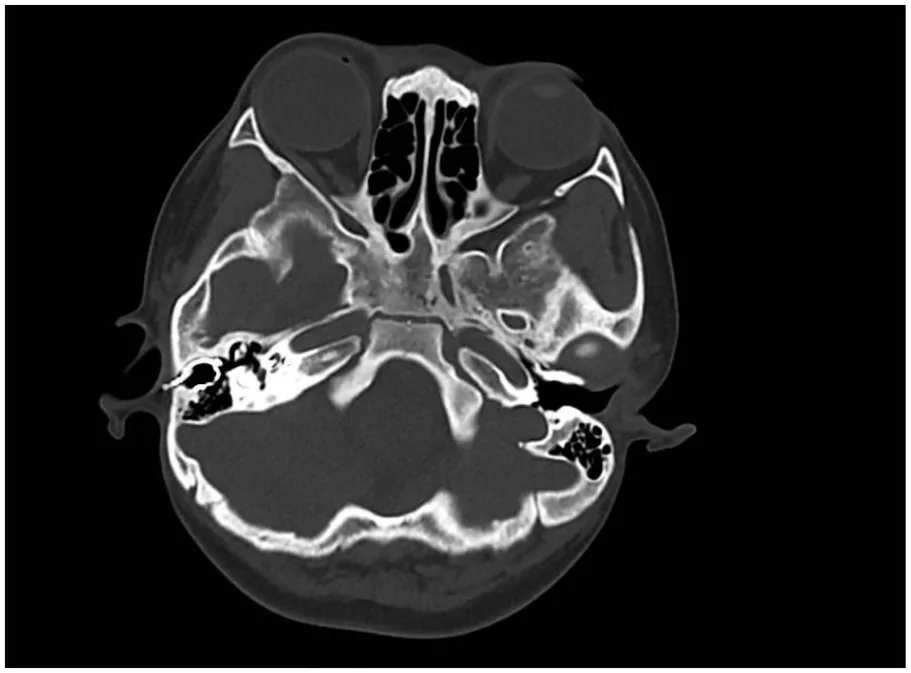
Brain MRI image of the patient.
